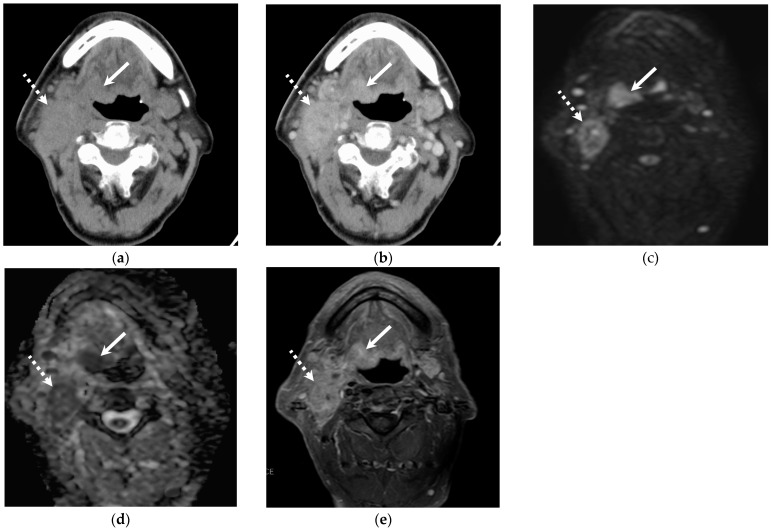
Representative images of an 86-year-old male with HPV-positive OPSCC in the base of the tongue. Local recurrence and pulmonary metastasis occurred. (a) Unenhanced CT shows soft tissue mass in the right base of the tongue (primary lesion, arrow) and right upper jugular lymph node metastasis (dotted arrow). CT attenuation of the nodal metastasis shows +42 HU. (b) Contrast-enhanced CT shows enhanced primary lesion (arrow) and nodal metastasis (dotted arrow). (c,d) Diffusion-weighted imaging and ADC map show high signal intensity and low ADC value in the primary lesion (arrow) and nodal metastasis (dotted arrow). (e) Fat-suppressed contrast-enhanced T1-weighted image shows primary lesion (arrow) and nodal metastasis (dotted arrow). The SIR of solid component is 2.15.
